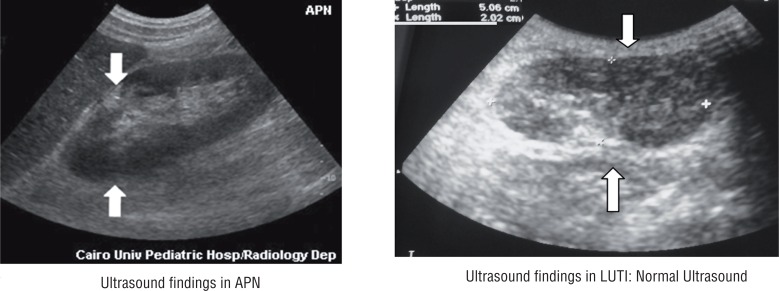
Ultrasound findings in the study cases.
